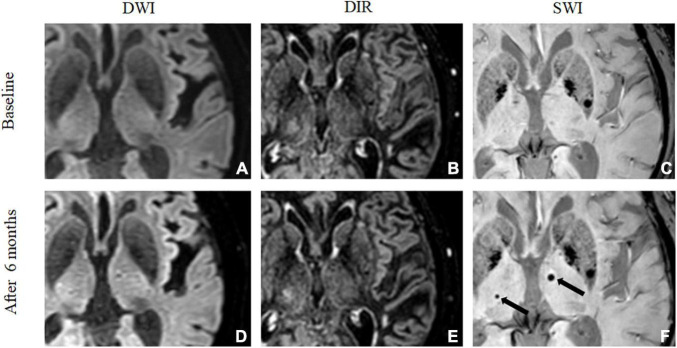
De novo* cerebral microbleeds mismatched with microinfarcts. A 73-year-old man showed no embolic microinfarct on DWI (A) or 3D-DIR (B), and no CMBs were found on SWI (C) at baseline. After 6 months, DWI (D) and 3D-DIR (E) did not detect any abnormalities. Two *de novo* CMBs were detected in the bilateral thalamus by SWI (F) after 6 months, but the CMBs were not related to the microinfarction.
